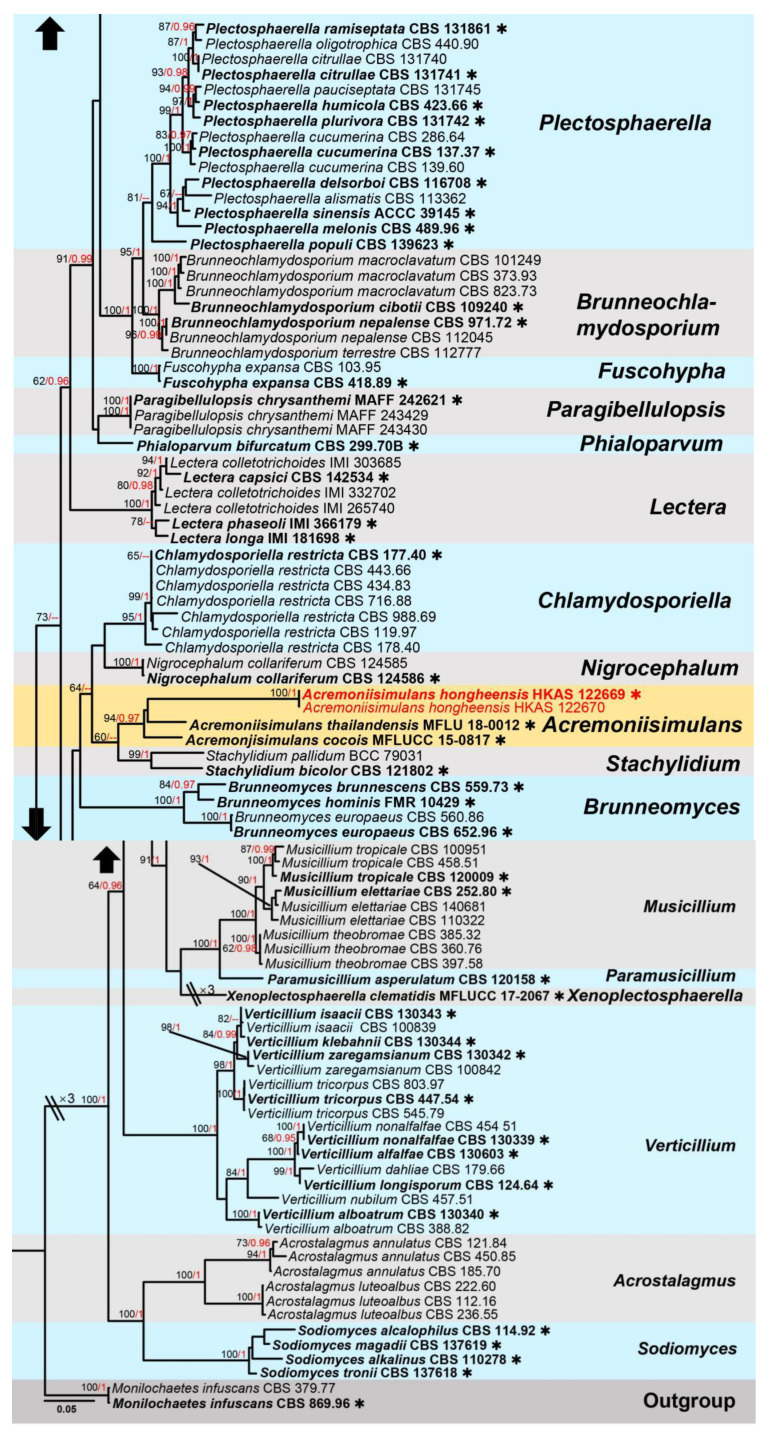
The phylogram based on a Maximum Likelihood analysis of combined LSU, ITS, *tef1*-*α* and *rpb2* sequence datasets. The analyzed gene contains 124 fungal strains and 2903 total characters including gaps (LSU: 1–833 bp, ITS: 834–1368 bp, *tef1*-*α*: 1369–2160 bp, *rpb2*: 2161–2903 bp). The tree topology of the ML resembles BI. The matrix had distinct alignment patterns, with the final ML optimization likelihood value of −32,895.306654 (ln). All free model parameters were estimated by the RAxML model, with 1112 distinct alignment patterns and 9.75% of undetermined characters or gaps. Estimated base frequencies were as follows: A = 0.225219, C = 0.295585, G = 0.280821 and T = 0.198375, with substitution rates AC = 0.822329, AG = 2.159486, AT = 1.120296, CG = 0.716819, CT = 5.475391 and GT = 1.000000. The gamma distribution shape parameter alpha = 0.656730, and the Tree-Length = 3.625234. The final average standard deviation of split frequencies at the end of total MCMC generations was calculated as 0.009897 in BI analysis. The type strains are denoted in bold with the symbol “✱” at the ends, and newly introduced species in this study are denoted in red. The nodes provide bootstrap values of at least 60% (ML, left) and Bayesian posterior probabilities of at least 0.95 (BI, right); hyphens (-) signify values that are less than 60% in ML and less than 0.95 in BI. The bluish and pale brown backgrounds were used to distinguish different genera in Plectosphaerellaceae, while the yellow background indicates the genus *Acremoniisimulans*.
